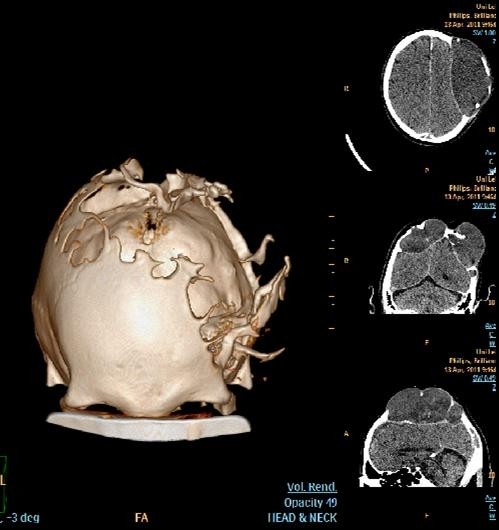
Volume rendering technique of the computed tomography (CT) scan in the bone intensity window and insets of axial, coronal, and sagittal computed tomography (CT) scans, show the calvarial defects, especially on the left side.
